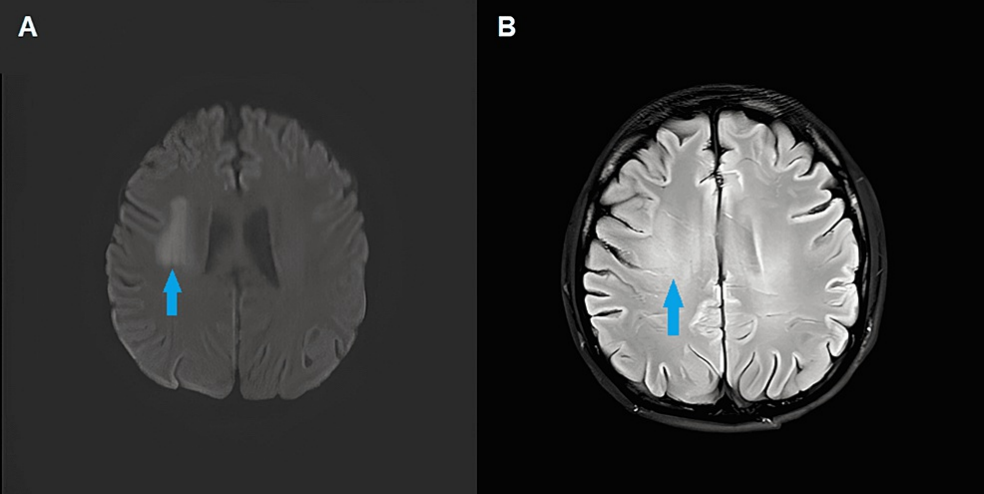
Brain MRI (A) Restricted diffusion on the diffuse-weighted image in the territory of the right middle cerebral artery. (B) FLAIR sequence with slight representation of the compromised territory without hemorrhagic transformation or contrast uptake. FLAIR, fluid-attenuated inversion recovery
